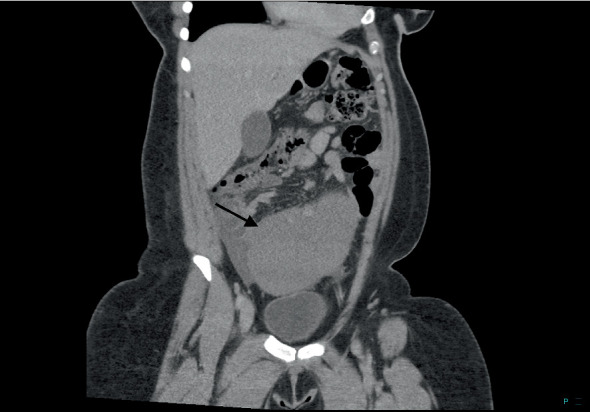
CT scan demonstrated the spleen located above the bladder rather than in the left upper quadrant.
